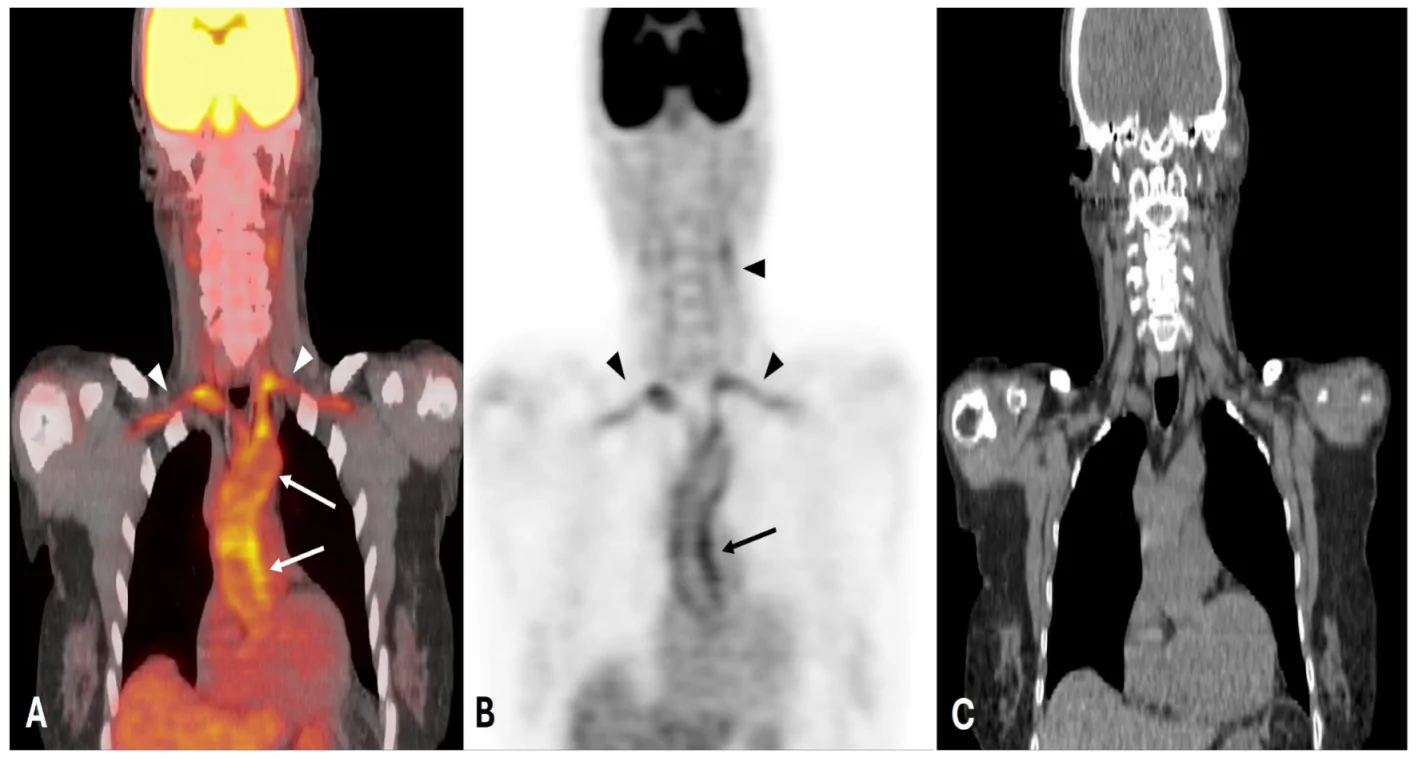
An example of a known giant cell arteritis. Coronal images of F-18 FDG PET/CT scan (A) fused, (B) AC PET,(C) CT without contrast showing intense tracer accumulation throughout the ascending aorta and aortic arch (arrows) as well as right brachiocephalic artery, bilateral subclavian arteries and carotid arteries (arrowheads) indicating active vasculitis.
